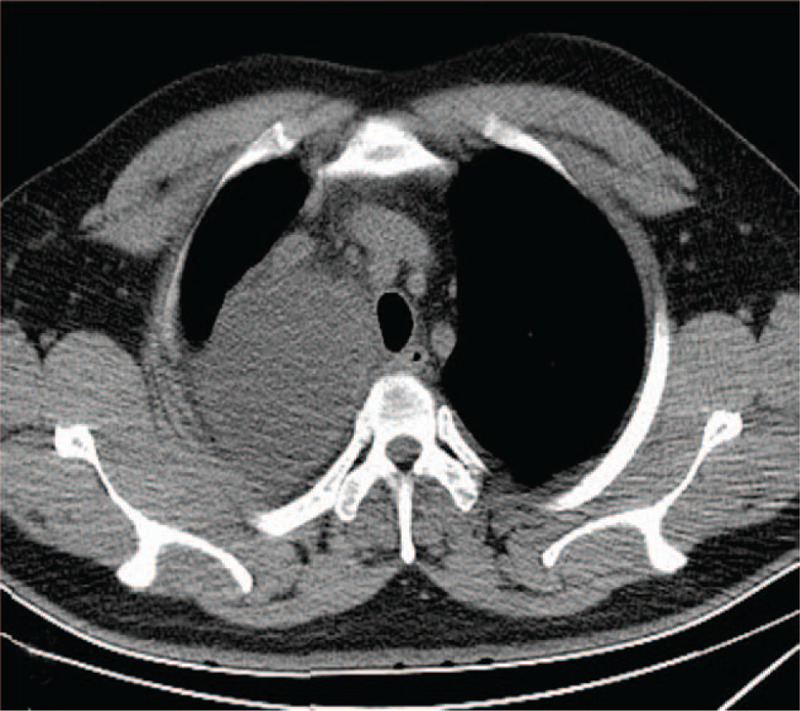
Chest CT on June 13 showed enlarged mediastinal cyst.
